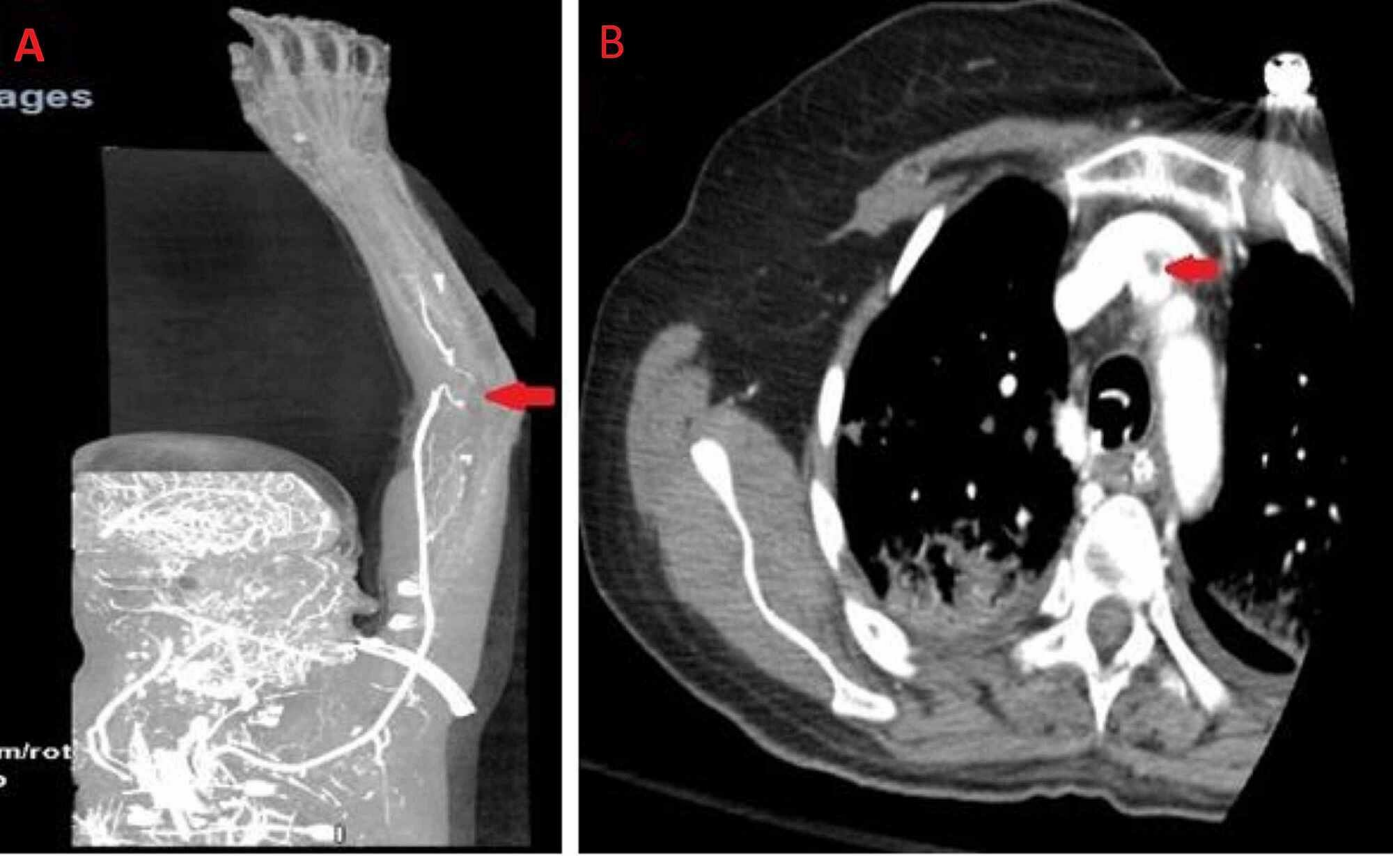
Computed tomography (CT) angiogram of right upper extremity showing occlusion of the most distal aspect of the right brachial artery at the bifurcation with no significant flow seen in the radial and ulnar arteries of the forearm (A) and small thrombus in the right innominate artery (B).
